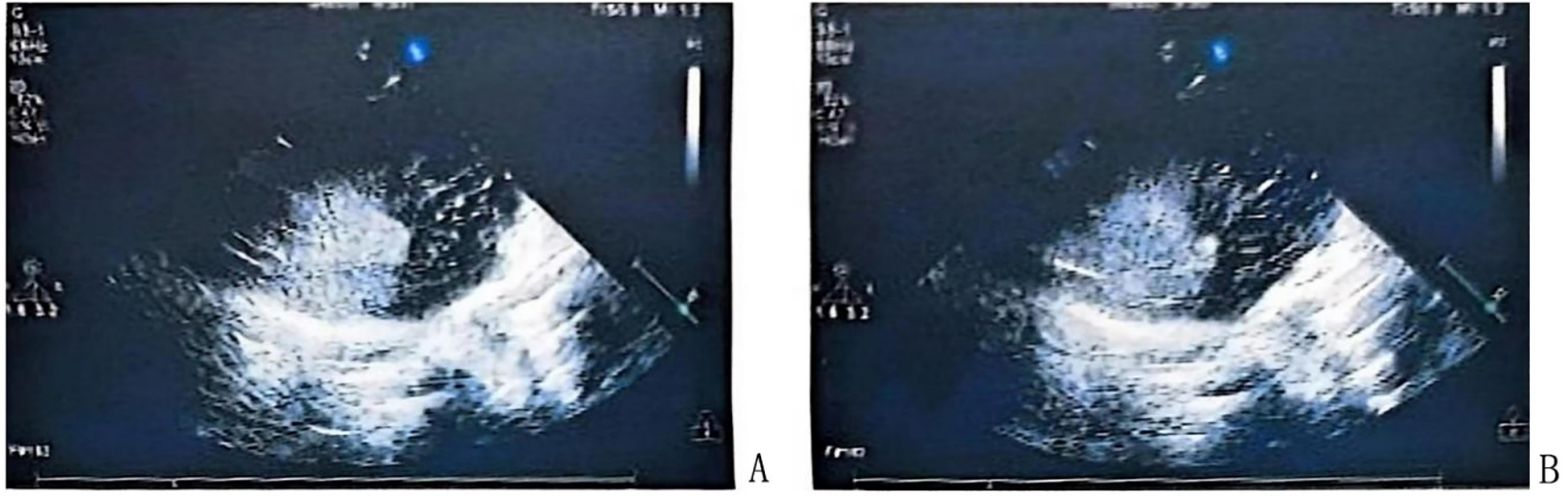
Saline contrast echocardiography in the apical four-chamber view (A,B) reveals abundant contrast microbubbles in the left atrium and left ventricle, respectively, confirming a large right-to-left shunt.
